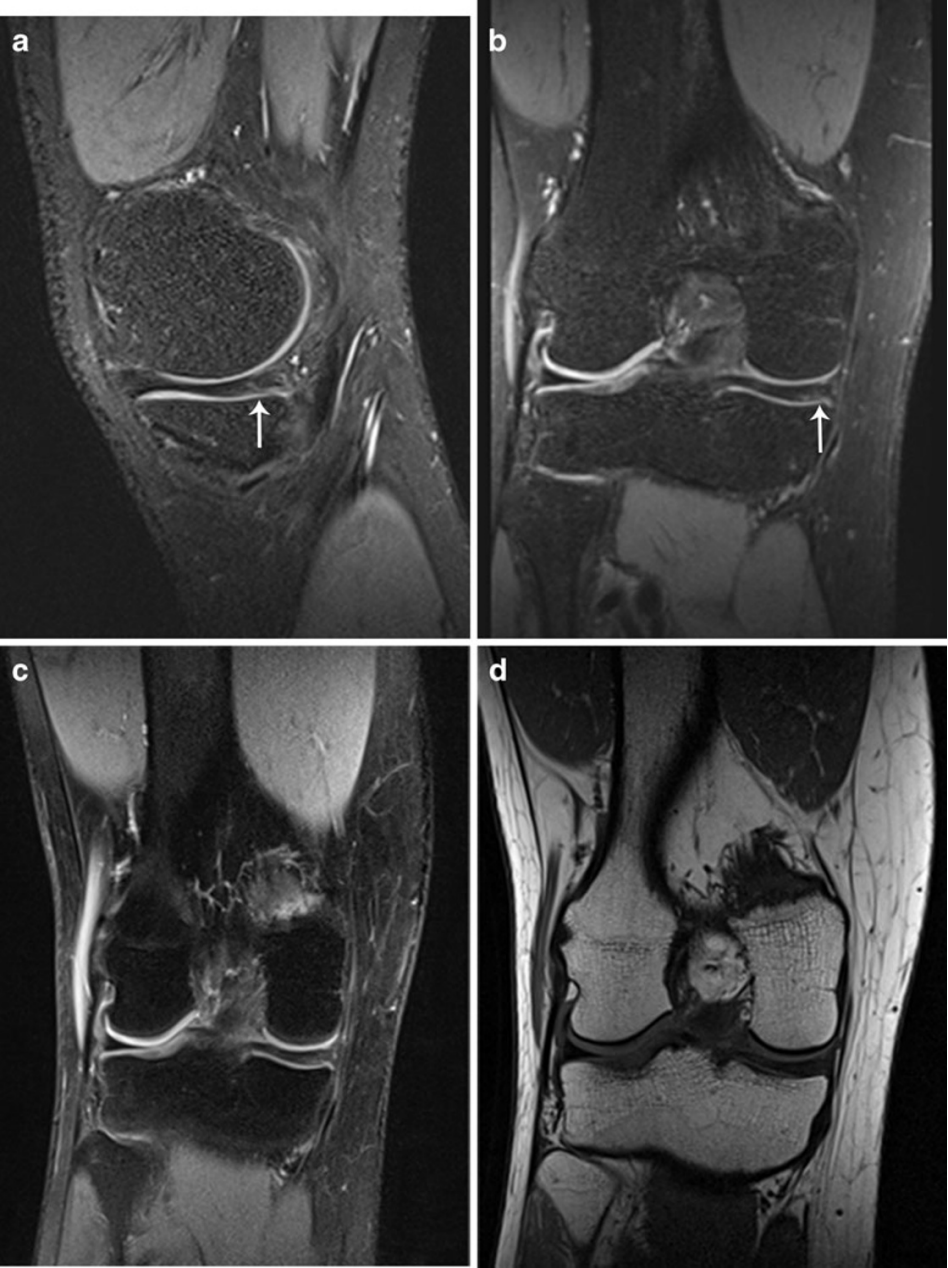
A 21-year-old male with a surgically confirmed normal medial meniscus, which was interpreted as a meniscal tear by all readers using 3D SPACE and as a normal meniscus by all readers using the routine MR protocol. Sagittal (a) and coronal (b) 3D SPACE images demonstrate grade 3 signal in the posterior horn of the medial meniscus extending to the inferior surface (*arrow*). Coronal 2D TSE intermediate-weighted FS (c) and T1-weighted (d) images show normal medial meniscus. Poor image contrast on 3D SPACE images was considered the primary cause of the discrepancy
